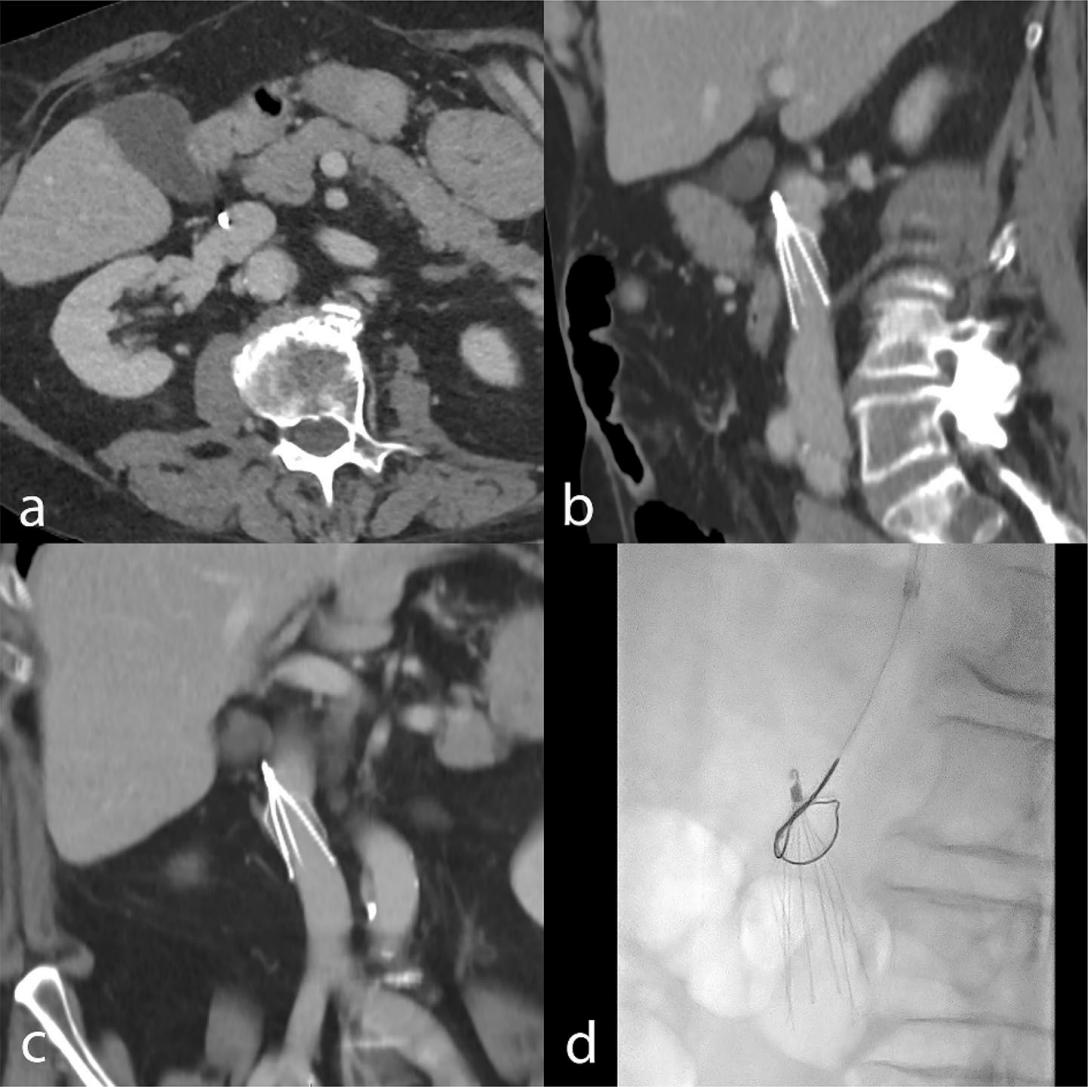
In a clinically eligible patient for IVCF retrieval, CT venography showed an embedded filter hook (a) due to a tilt visible both on sagittal (b) and coronal (c) reformatted images. Fluoroscopy image during the procedure shows unsuccessful attempt to retrieve the IVCF using a single-loop snare system (d). *CT* computed tomography, *IVCF* inferior vena cava filter.
